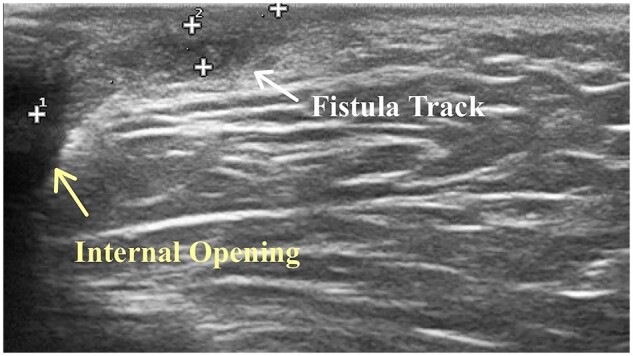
Ultrasonic manifestations of anal fistula. The yellow arrow marks the internal opening and the white arrow with “+” symbols marks the edge of the fistula track
